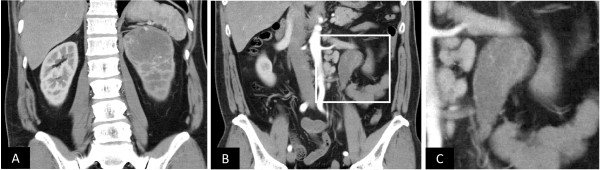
Coronal section of preoperative abdominal CT. A solid tumor in the upper pole of the left kidney (A) and the tumor thrombus (B) showed early-phase contrast enhancement.
